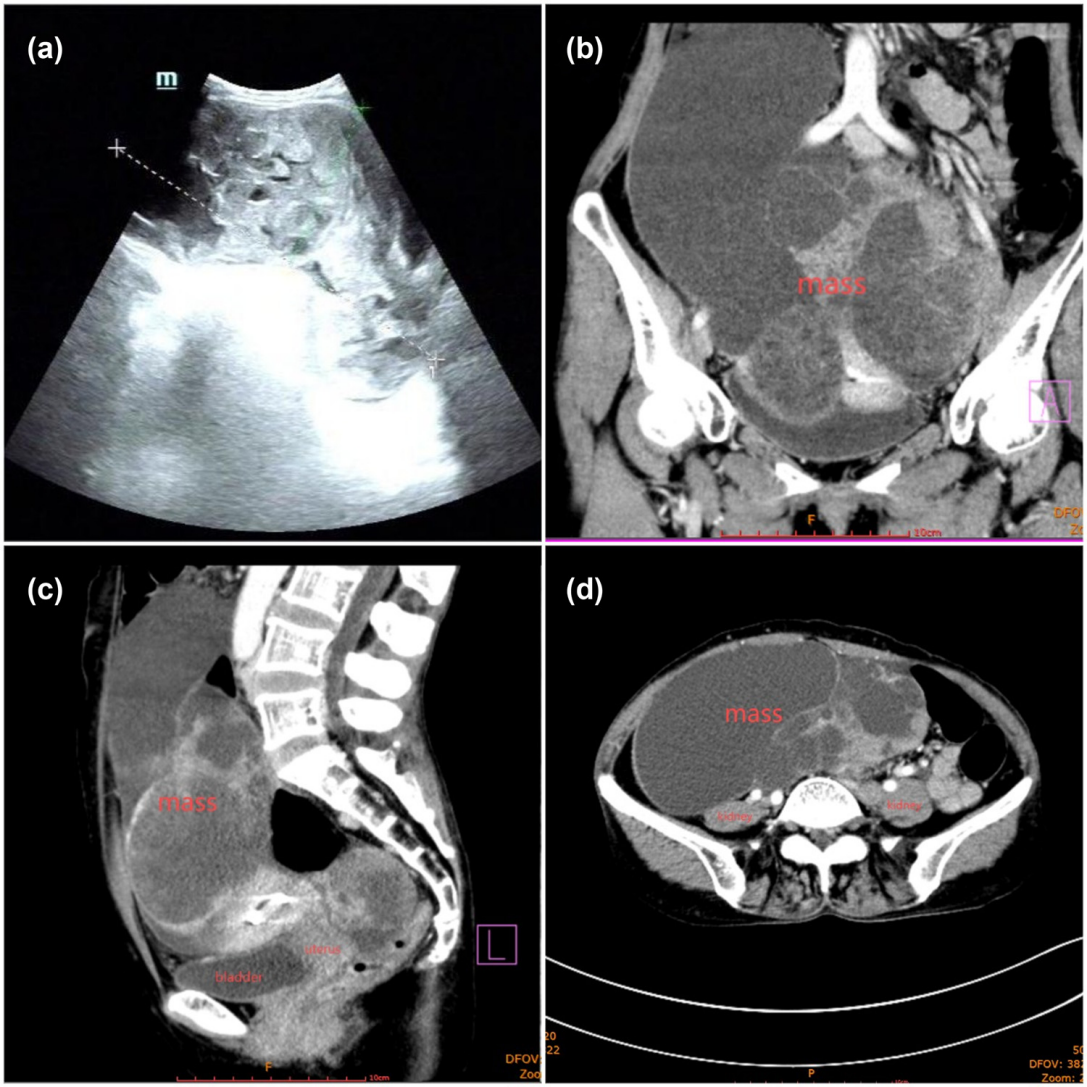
B-ultrasound and CT image of the patient. (a) B-ultrasound showed a multilocular, mixed solid, and cystic mass. (b)–(d) CT scan revealed a 133 mm × 229 mm mass located in the pelvic and abdominal cavity; it was closely adhered to the uterus, the upper bladder wall, and the anterior rectal wall, with unclear demarcation. The mass was solid and cystic, with septations and obvious enhancement; there were no signs of pelvic effusion, and no swollen lymph nodes were observed in the abdominopelvic cavity.
